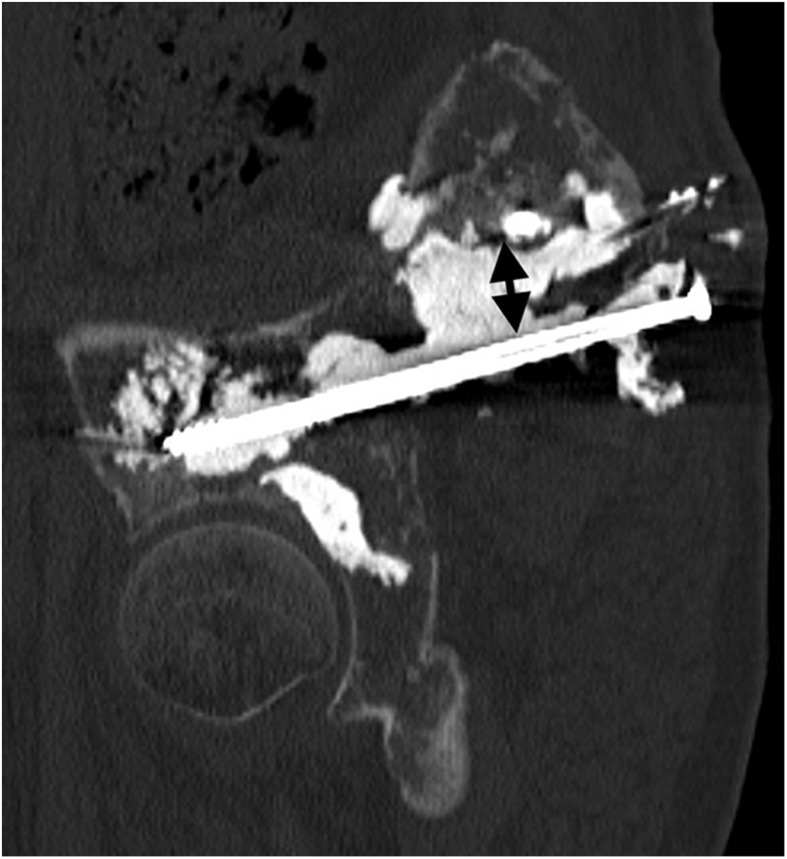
Oblique sagittal reformatted view of the final post-procedure CT (bone window, slice thickness 1 mm) of the pelvic bone shows a continuous cement bridge between the iliac screw and the cross section of the proximal part of the sacral screw (double black arrow).
